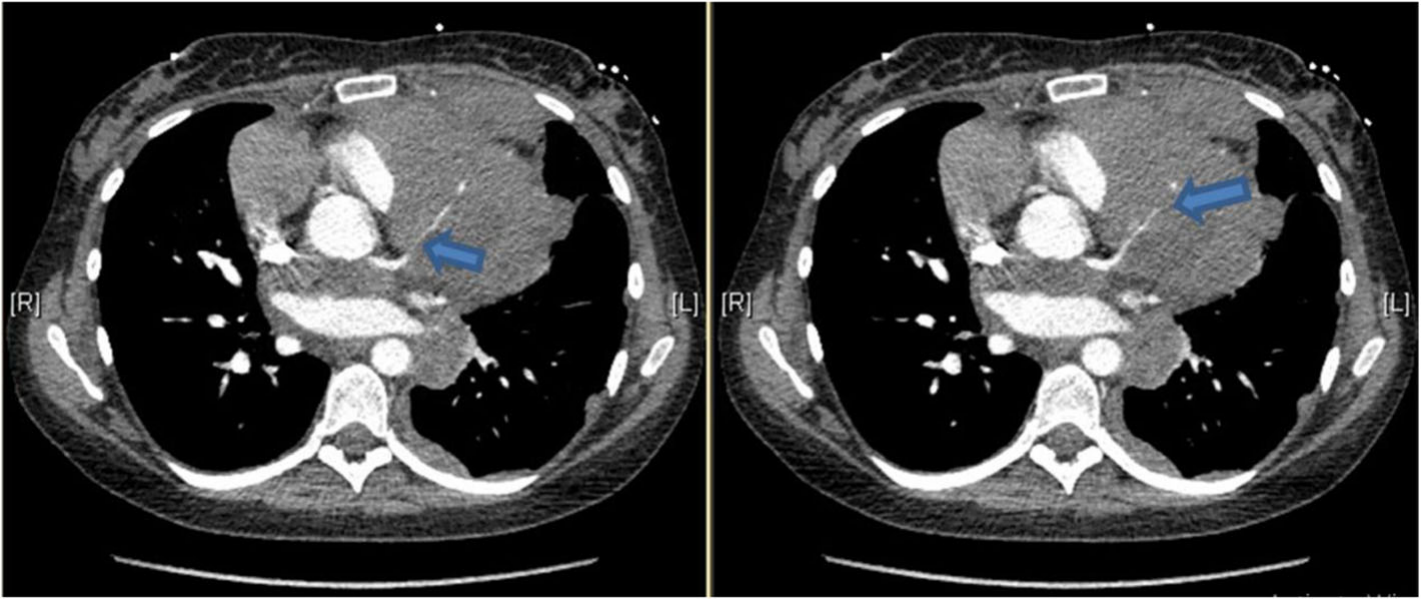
CT pulmonary angiogram reveals encasement and displacement of the left anterior descending coronary artery (blue arrows)
